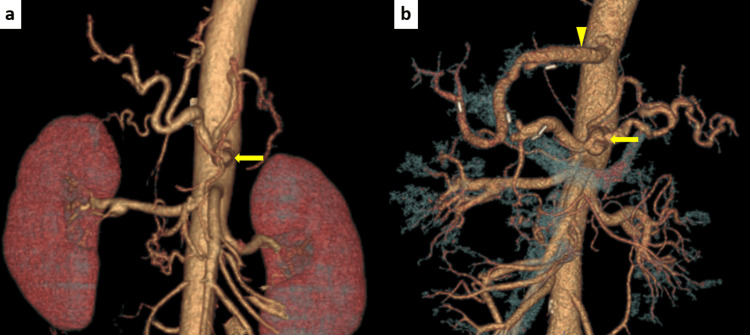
3D-computed tomography angiogram (a) Before liver transplant and (b) After transplant The iliac artery conduit was patent (arrowhead), and the size of the celiac artery aneurysm was not changed (arrow).
